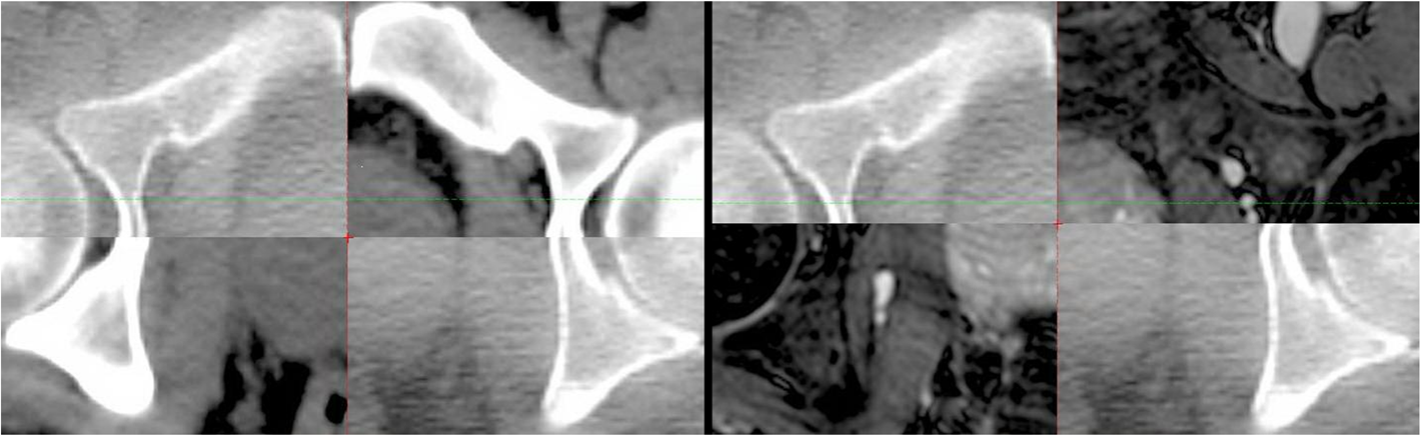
Registration overlays of CBCT with either CT (on the left) or MRI (on the right).
